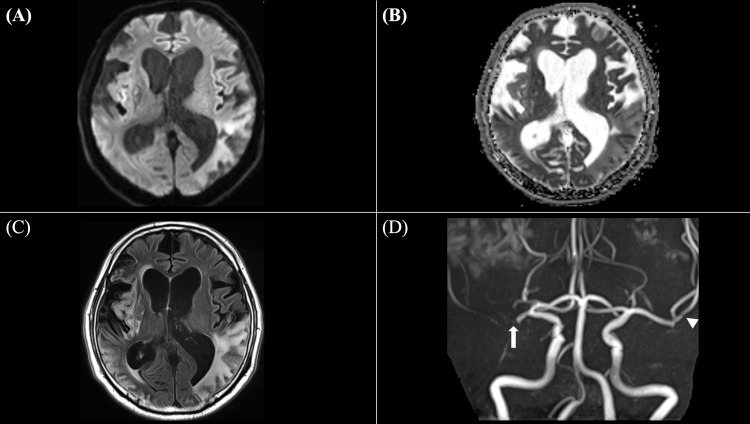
Follow-up MRI findings on hospital day 21 A: diffusion-weighted imaging (DWI) reveals no new hyperintense lesions, confirming the absence of new ischemic events and indicating stable infarct evolution; B: apparent diffusion coefficient (ADC) map shows no newly decreased ADC values, while previously restricted areas demonstrate ADC elevation, indicating subacute infarct evolution; C: fluid-attenuated inversion recovery (FLAIR) imaging demonstrates persistent vasogenic edema in the cortical infarction areas, without new signal abnormalities; D: time-of-flight (TOF) magnetic resonance angiography (MRA) shows persistent right middle cerebral artery (MCA) occlusion (arrow) and persistent left MCA stenosis (arrowhead), with no improvement of vascular patency.
